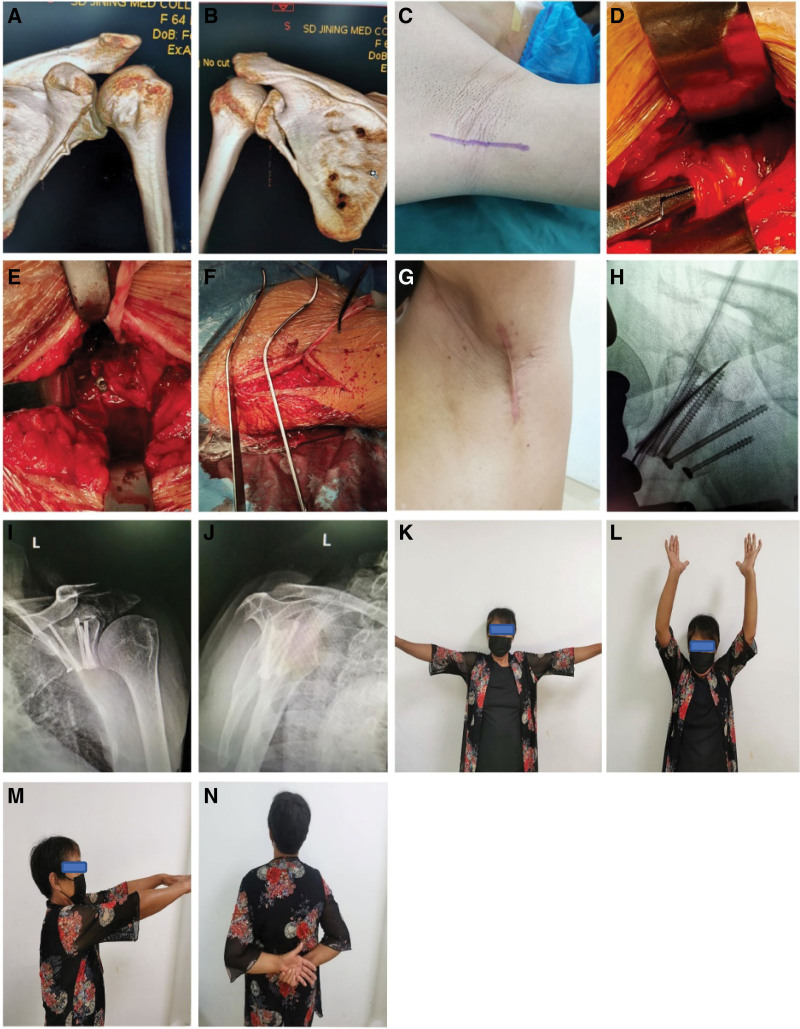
Female patient, 64 years old, was hit by a car while riding her electric bicycle. Preoperative CT 3D reconstruction (A, B) shows an Ideberg II scapular glenoid fracture with the scapular glenoid fracture line extending to the lateral edge of the scapula. The operation was treated by axillary approach with general anesthesia in the supine position, the incision was made from the apex of the axilla along the anterior border of the latissimus dorsi muscle towards the proximal end of the trunk to the level of the subscapular angle (C), and the axillary nerve and the posterior spinohumeral artery were revealed (D). The glenohumeral capsule is further exposed by blunt inward separation along the anterior border of the latissimus dorsi muscle gap, and the fracture is subsequently repositioned and fixed with screws (E). The Hohmann pulling hook was intraoperatively used to pull the muscle (F). Intraoperative X-ray fluoroscopy reveals a well-positioned scaphoid fracture with a hollow screw (H). The incision is closed with sutures, approximately 7 cm long (G), and the incision can be obscured when the affected limb drops. Positive and lateral x-rays of the scapula of the shoulder joint 3 months after surgery showed that the scapular pelvis fracture was well reset (I, J), and the patient’s shoulder function returned to normal (K–N).
